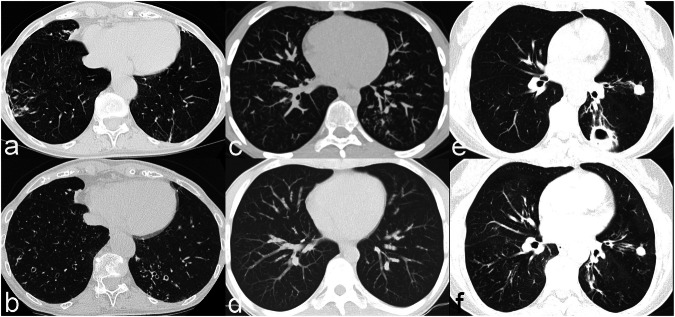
Serial CT in three patients with nontuberculous PD. Patient 1 is a 78-year-old woman with *M. avium* infection. CT shows progressive bronchiectasis between BL (a) and FU (b) CT 2 years later without antibiotic treatment and persistent cultures. Patient 2 is a 19-year-old male patient with immunosuppression and *M. kansasii* infection. At BL CT (c) there were bronchiolitic changes in CT, which disappeared completely in the FU CT (d) 1.5 years later after successful antibiotic treatment. Patient 3 is a 60-year-old woman with *M. avium* infection. Cavitary disease in BL-CT (e) disappeared in FU CT (f) 4 years later after successful antibiotic treatment, whereas nodules persisted
